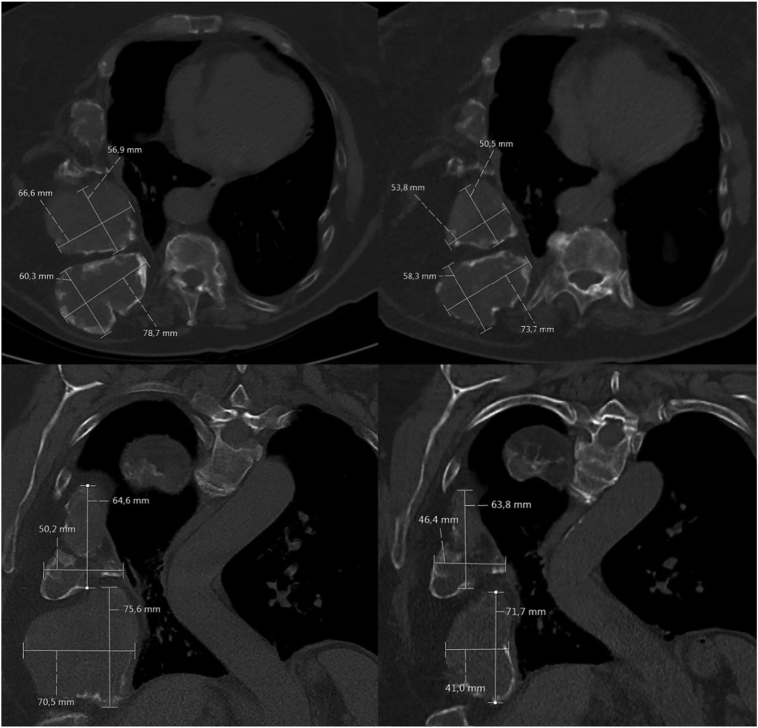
Regression of rib lesions before denosumab treatment (left, upper row: axial plane, lower row: coronal plane) and after 3.5 denosumab treatment (right: upper row: axial plane, lower row: coronal plane) on chest CT-scan of case 2.
